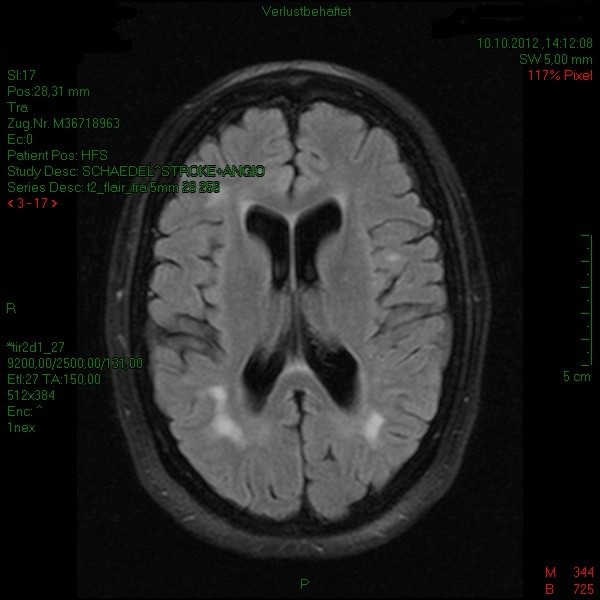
T2-weighted cerebral MRI scan. There are confluent white matter lesions in all cerebral lobes and signs of brain atrophy.
